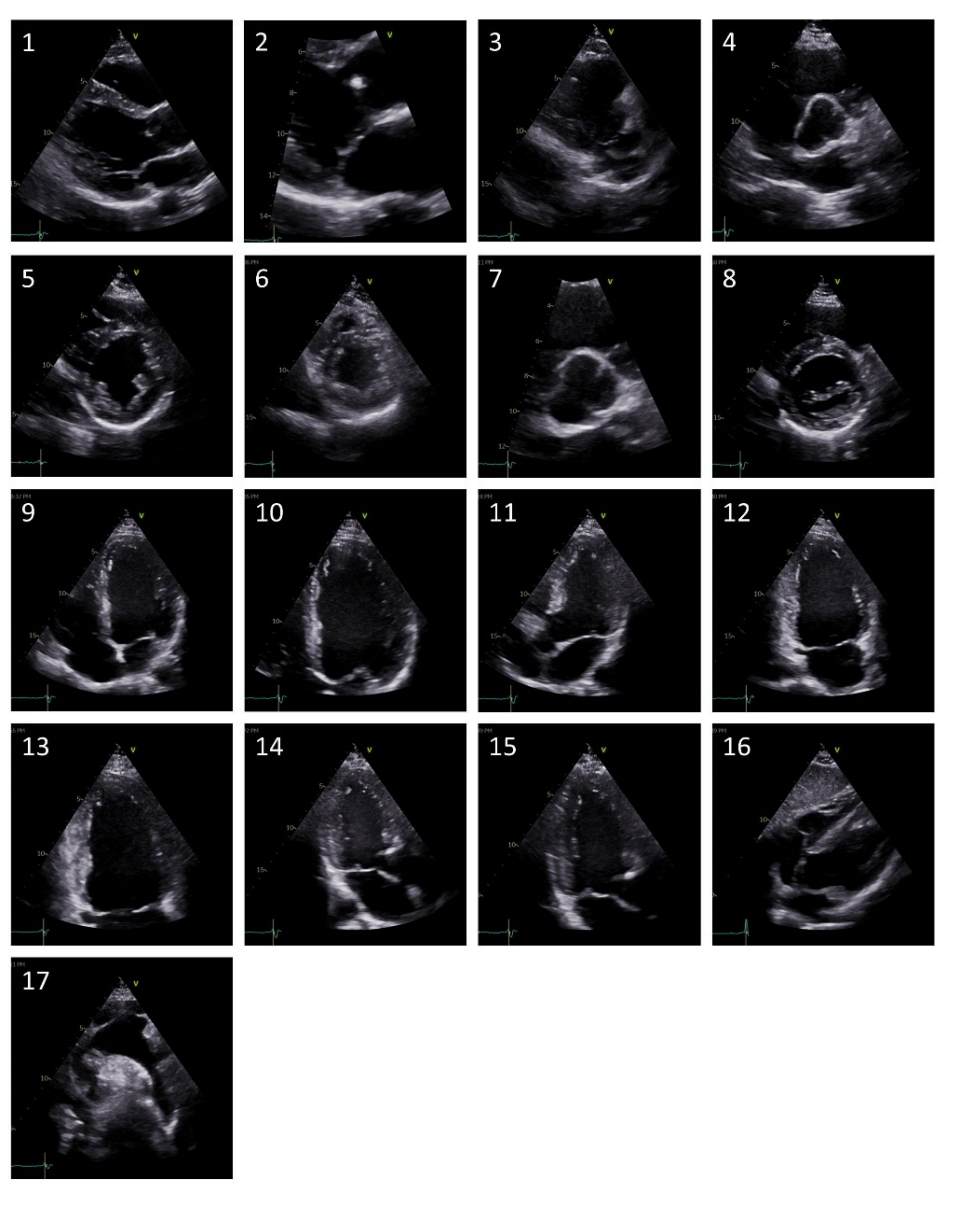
Representative images of the included seventeen separate echocardiographic views. (1) = Parasternal long axis (PLAX) left ventricle, (2) = PLAX zoomed MV, (3) = PLAX RV inflow, (4) = Parasternal short axis (PSAX) focus on AV, (5) = PSAX papillary muscles, (6) = PSAX apex, (7) = PSAX zoomed AV, (8) = PSAX MV, (9) = Apical 4 chamber (A4C), (10) = A4C zoomed left ventricle, (11) = Apical 5 chamber, (12) = Apical 2 chamber (A2C), (13) = A2C zoomed left ventricle, (14) = Apical 3 chamber (A3C), (15) = A3C zoomed left ventricle, (16) = Subcostal 4 chamber, (17) = Suprasternal aortic arch.
